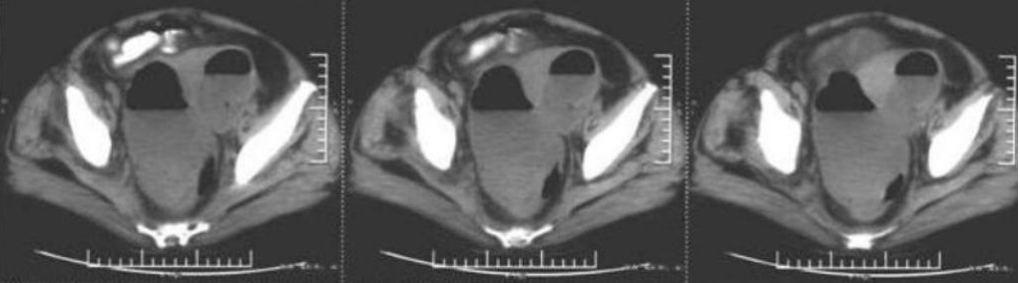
Computed tomography scan of pelvis showing a large local air-fluid level.
